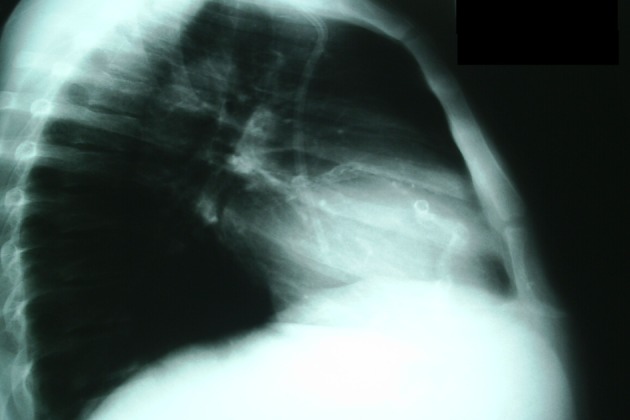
Chest radiography showing calcified right coronary and aortic valve.
